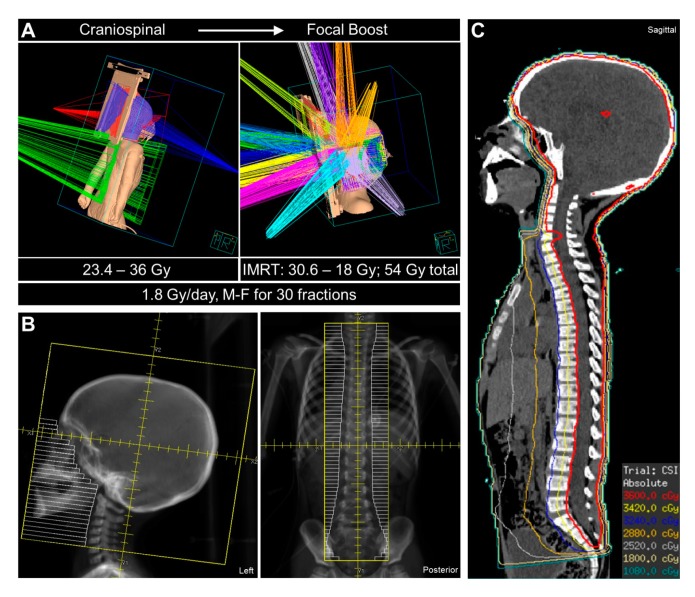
Clinical radiation therapy for medulloblastoma. (A) Three-dimensional rendering of craniospinal irradiation (CSI) (left) in a pediatric patient with medulloblastoma treated in the supine position with parallel-opposed lateral beams (red, purple) and a single posterior-to-anterior (PA) beam (green) and with intensity-modulated radiation therapy (IMRT)-based primary tumor site boost irradiation with multiple beams (right). Typical CSI and boost doses and fractionation patterns are noted. M–F, Monday through Friday. (B) Beam’s eye view of the cranium (left) and spine (right) CSI fields on digitally reconstructed radiographs (DRRs), with multileaf collimators depicted in white. (C) CSI isodose distributions overlaid on the sagittal planning computed tomography (CT) scan.
